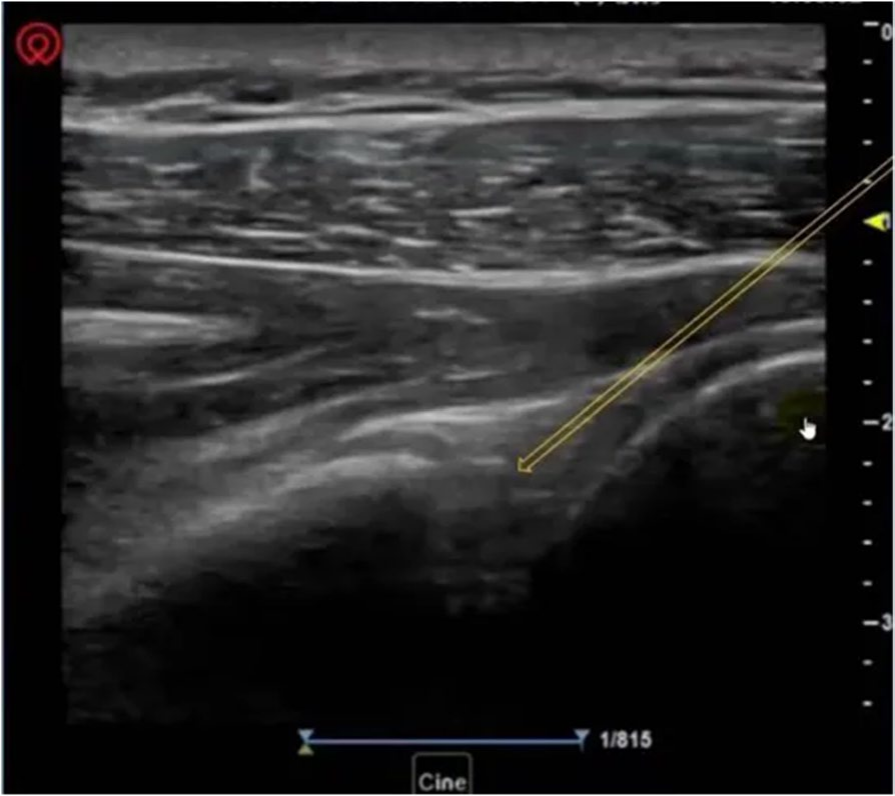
Ultrasound-guided intra-articular injection of shoulder
